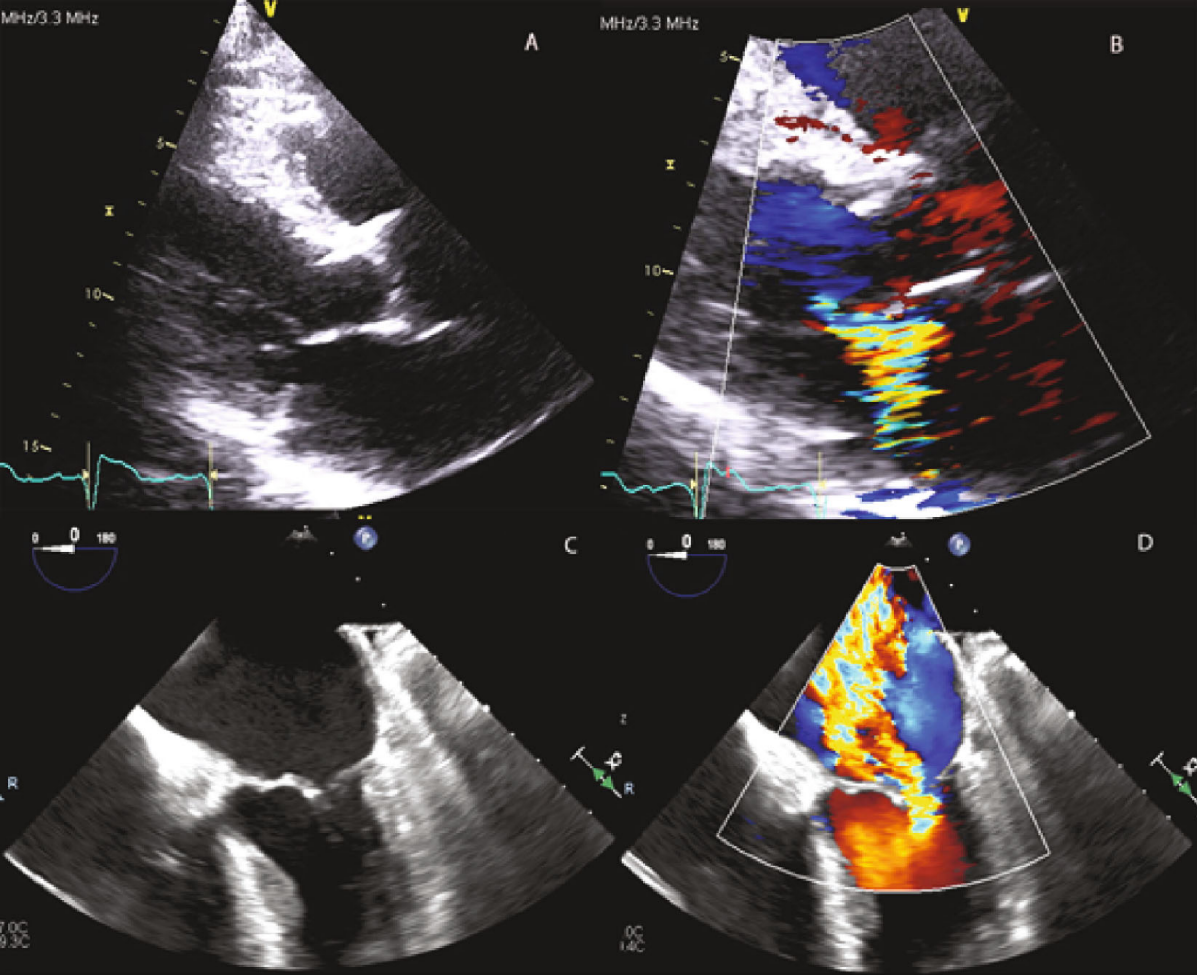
Transthoracic and transesophageal echocardiograms after leadless pacemaker insertion. (a) A transthoracic echocardiogram (parasternal long) taken after insertion of the leadless pacemaker showing incomplete coaptation of mitral valve leaflets. (b) A transthoracic echocardiogram with color (parasternal long) showing severe mitral regurgitation (effective regurgitant orifice area of 0.51 cm^2^ and regurgitant volume of 52 ml) after leadless pacemaker insertion. (c) A transesophageal echocardiogram showing incomplete coaptation of mitral valve leaflets following leadless pacemaker implantation. (d) A transesophageal echocardiogram with color showing severe mitral regurgitation following leadless pacemaker implantation.
